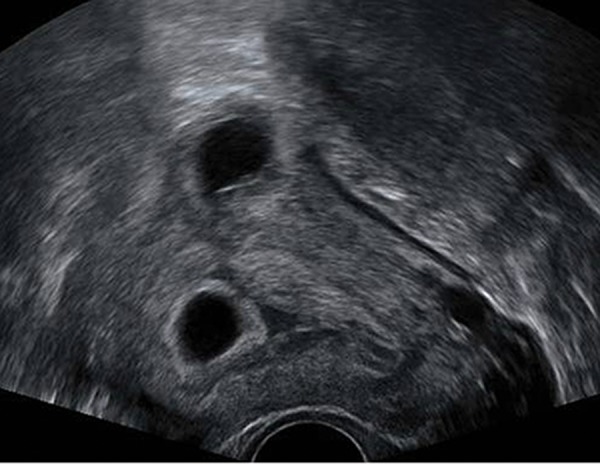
Transvaginal ultrasonography showed the gestational sacs
